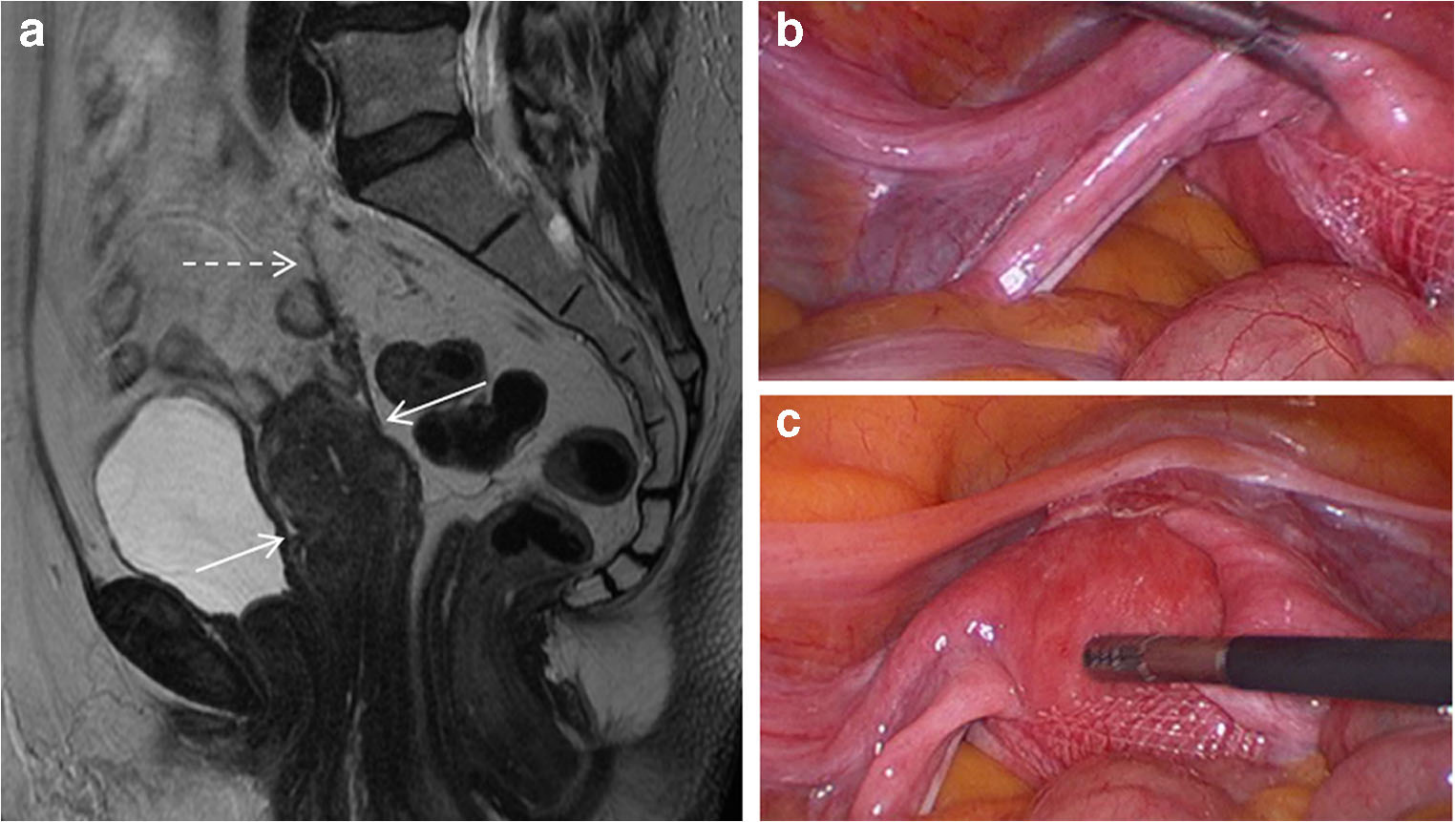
MR scan (A) and corresponding laparoscopic images (B and C) of uterine compression by sacrohysteropexy mesh. A Sagittal MRI showing compression of the uterus (two solid arrows) with uterine prolapse with intact but slightly lax mesh (dashed arrow). B and C Corresponding laparoscopic images for the same patient which shows the uterus prolapsed through the arms of the sacrohysteropexy mesh
